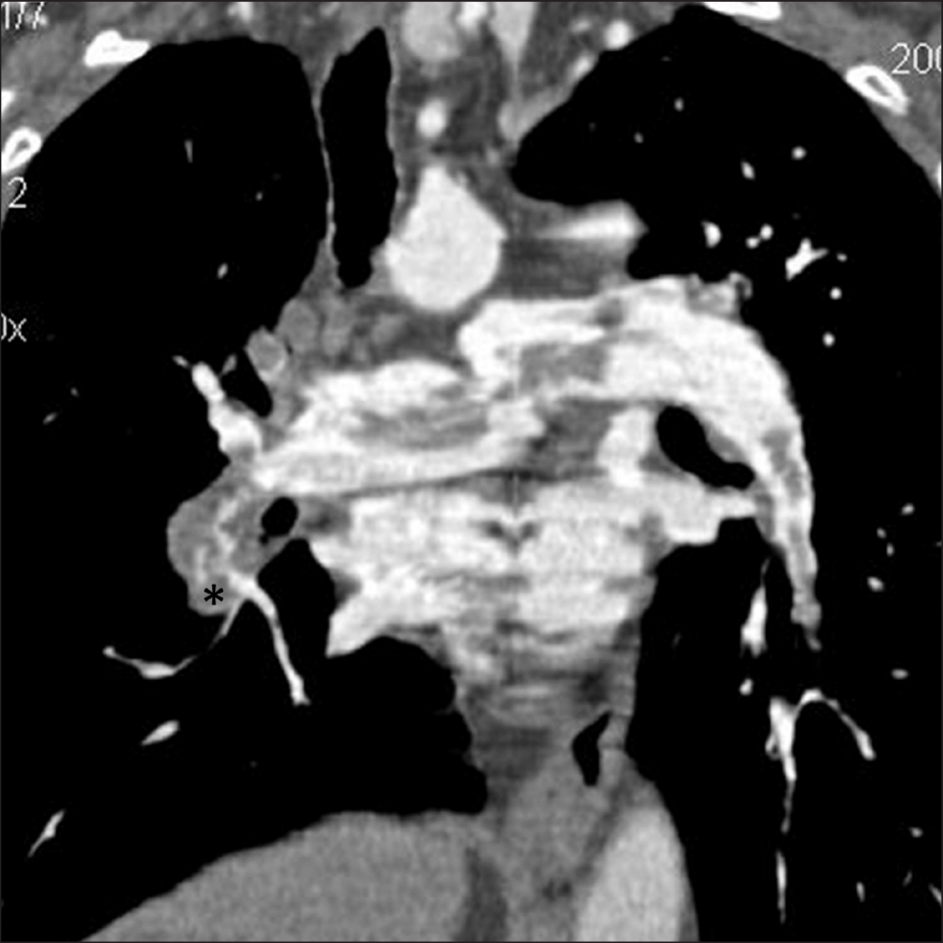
Coronal contrast-enhanced CT pulmonary angiography image demonstrating emboli filled and enlarged right intermediate pulmonary artery. The pulmonary artery abruptly tapers with due to the blood clot, whose distal end is marked (star).
